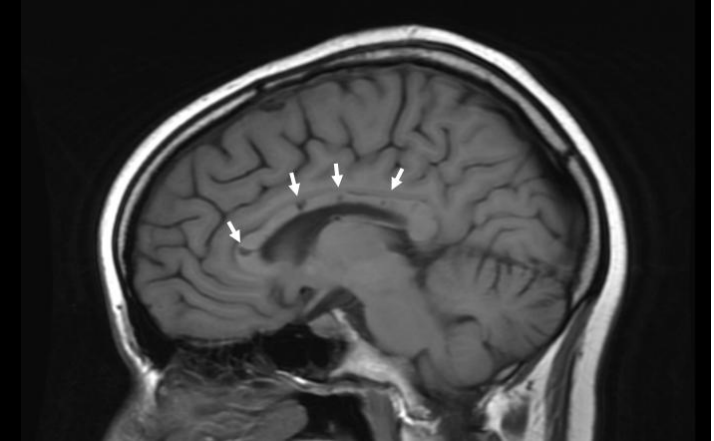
MRI brain. Sagittal T1 image showing the pathognomonic central callosal "holes" (microinfarcts) of SS. These residual "holes" (and sometimes, "spokes") develop as the acute callosal changes resolve.
